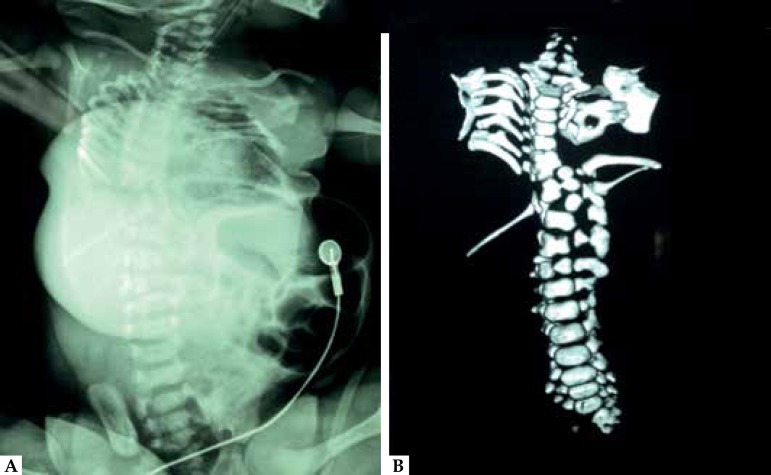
Spinal radiographs (A) and 3D computed tomography
